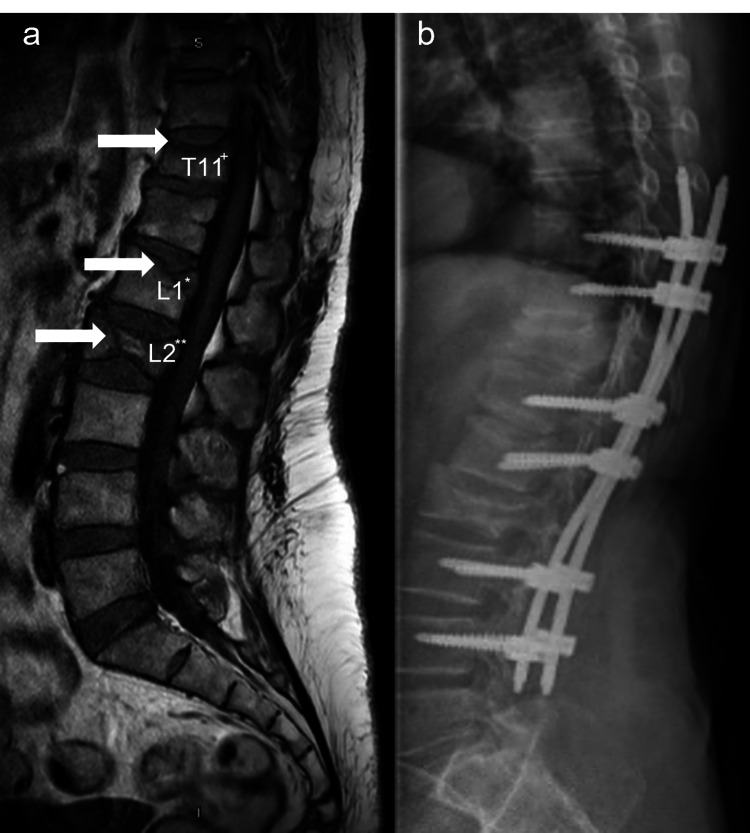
Magnetic resonance imaging of spinal fractures caused by generalized tetanus. a) Spinal fractures caused by progressive tetanus. b) Lumbar osteosynthesis performed at T11, L1 and L2. +: mild fracture of T11. *: annular fracture of the L1 plateau. : burst fractures in L2.
